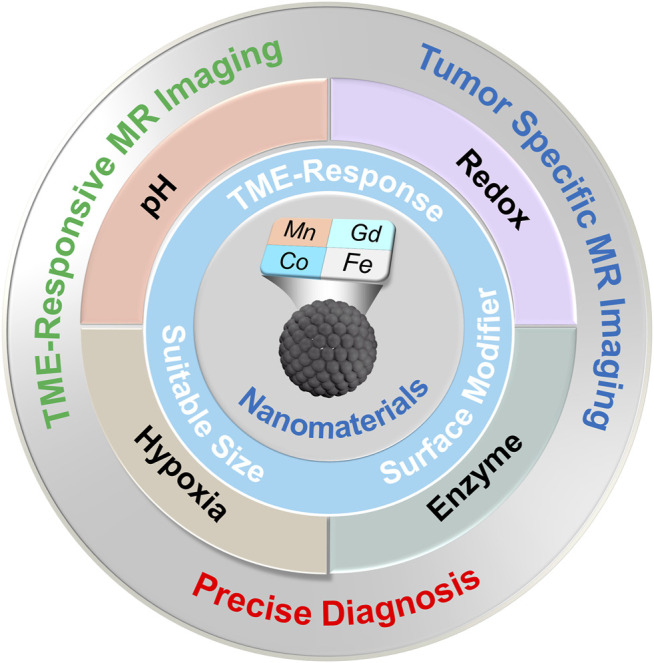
General strategies to synthesize nanotheranostic agents for TME-responsive MRI.
